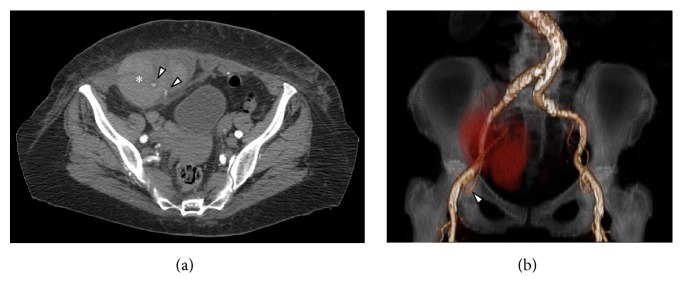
(a) MD-CT axial view demonstrates the presence of a haematoma into the right rectus sheath (*∗*) with two spots of active bleeding from distal collaterals of the right inferior epigastric artery (arrowheads). (b) MD-CT coronal Volume Rendering Technique image with right inferior epigastric artery reconstruction (arrowhead) and the haematoma into the right rectus sheath as a red shadow.
